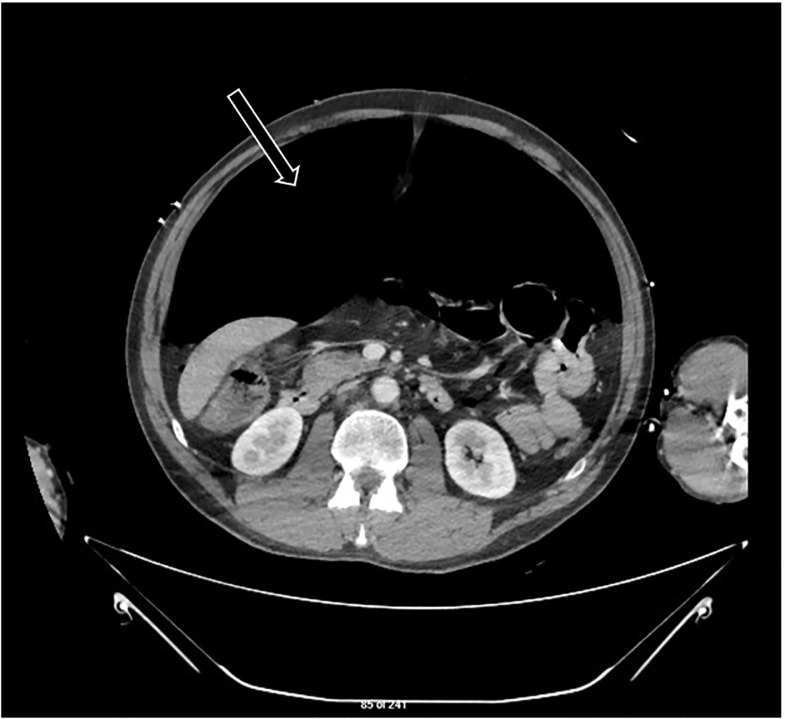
Computed tomography of the abdomen with intravenous contrast at the level of the inferior tip of the liver and kidneys showing large pneumoperitoneum, as noted by arrow, with tension physiology.
